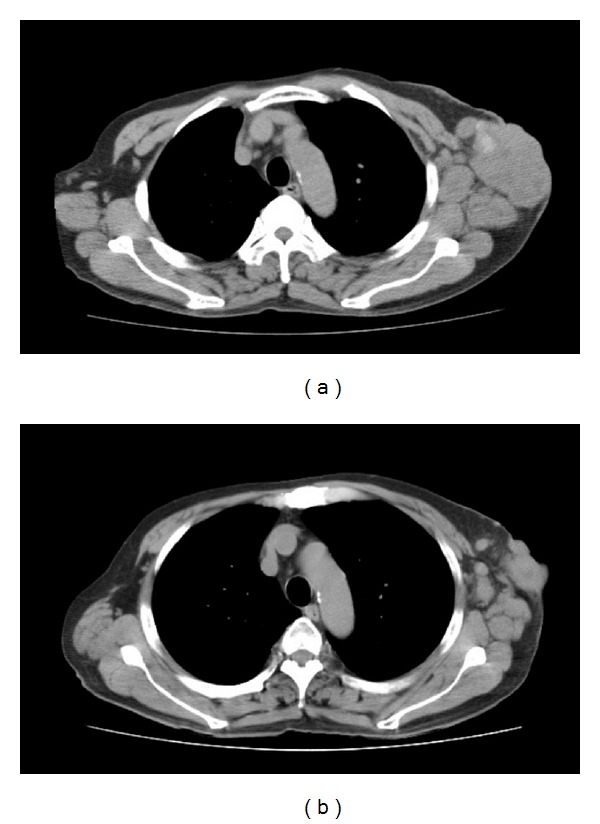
(a) Computed tomography (CT) showed an exposed and lobulated 77 mm soft tissue density mass with faint calcification in wide contact with the skin, enlarged lymph nodes in the left axilla, and small round lymph nodes less than 10 mm in the mediastinum. (b) After the neoadjuvant chemotherapy, the tumor was reduced to 51 mm in diameter, while the small mediastinum lymph nodes remained about the same in size.
